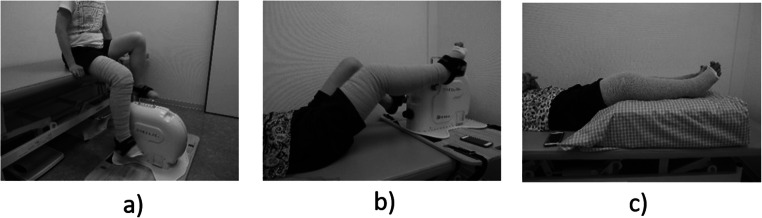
a Seated AECT is performed on a bicycle ergometer while wearing elastic compression bandages on the affected limb. b Supine AECT is performed on a bicycle ergometer while wearing elastic compression bandages on the affected limb. c T is performed with elevation of both legs while wearing elastic compression bandages on the affected limb. *AECT, active exercise with compression therapy; seated AECT, AECT in a seated position; supine AECT, AECT in a supine position; CT, compression-only therapy
